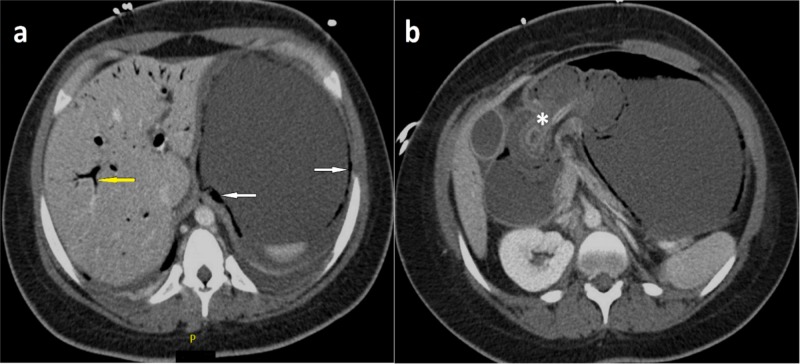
Axial plane computed tomography of the abdomen and pelvis (with contrast) at two different levels (T12: a and L1: b) showing percutaneous endoscopic gastrostomy tube displacement into the proximal duodenum (asterisk) with evidence of portal vein gas (yellow arrow) and pneumatosis (white arrows).
